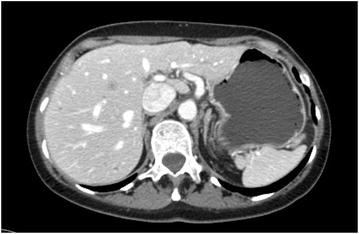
New hepatic lesion. In October 2012, CT scan showed liver progression for the appearance of a new lesion
